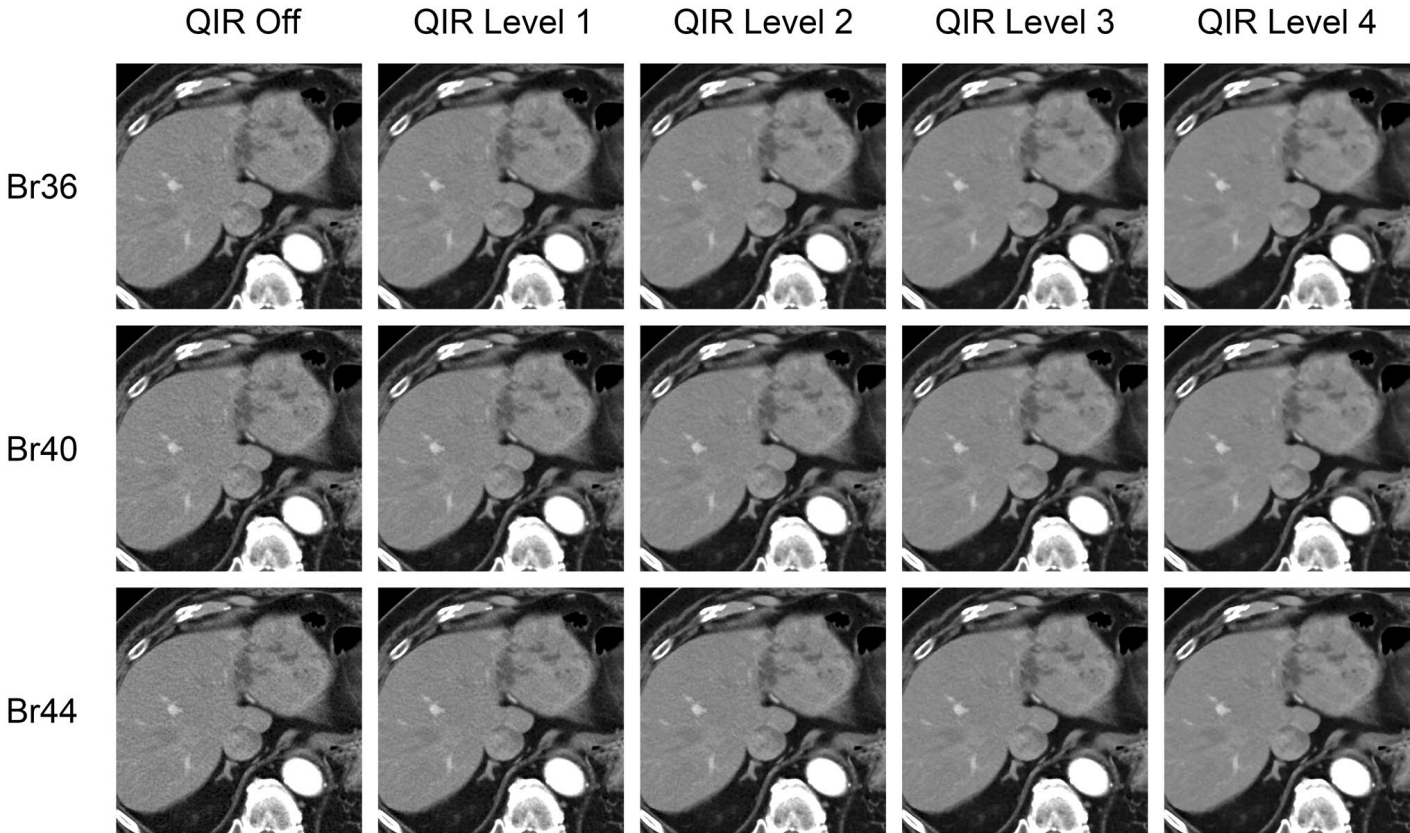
Photon-counting CT images with different reconstruction settings are shown aligned. The higher the resolution index (Br36, Br40, Br44), the higher the resolution, but the higher the image noise. The higher the level of the Quantum Iterative Reconstruction (QIR) algorithm, the lower the image noise
